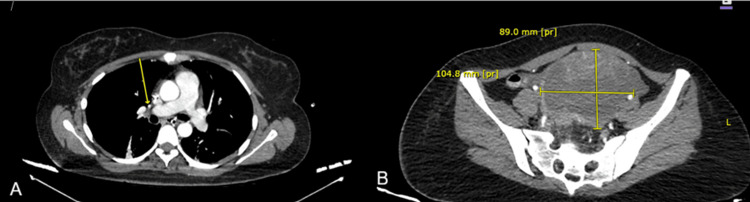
Right pulmonary thromboembolism and pelvic mass (A) Axial chest computed tomography (CT) showing a filling defect in the right pulmonary artery (arrow), consistent with pulmonary thromboembolism. (B) Axial pelvic CT revealing a large pelvic mass of indeterminate etiology.
